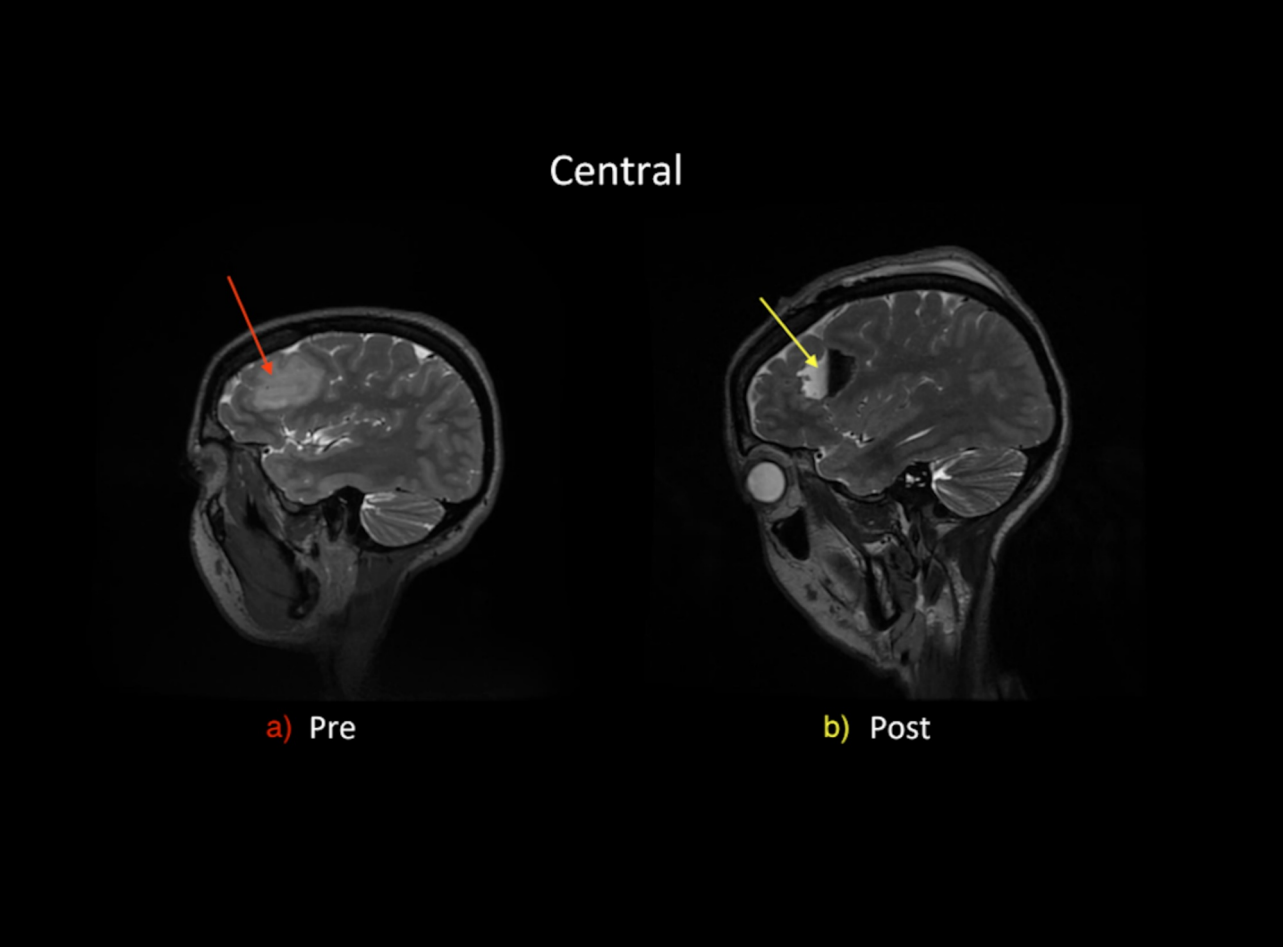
Central view of (a) preoperative MRI and (b) postoperative MRI. (a) The preoperative MRI shows tumor, 3.1 x 3.2 cm, in the posterior inferior portion of the left frontal lobe. (b) The postoperative MRI shows postoperative changes as fluid-filled resection cavity, 4.5 x 4 cm, in the posterior inferior portion of the left frontal lobe.
